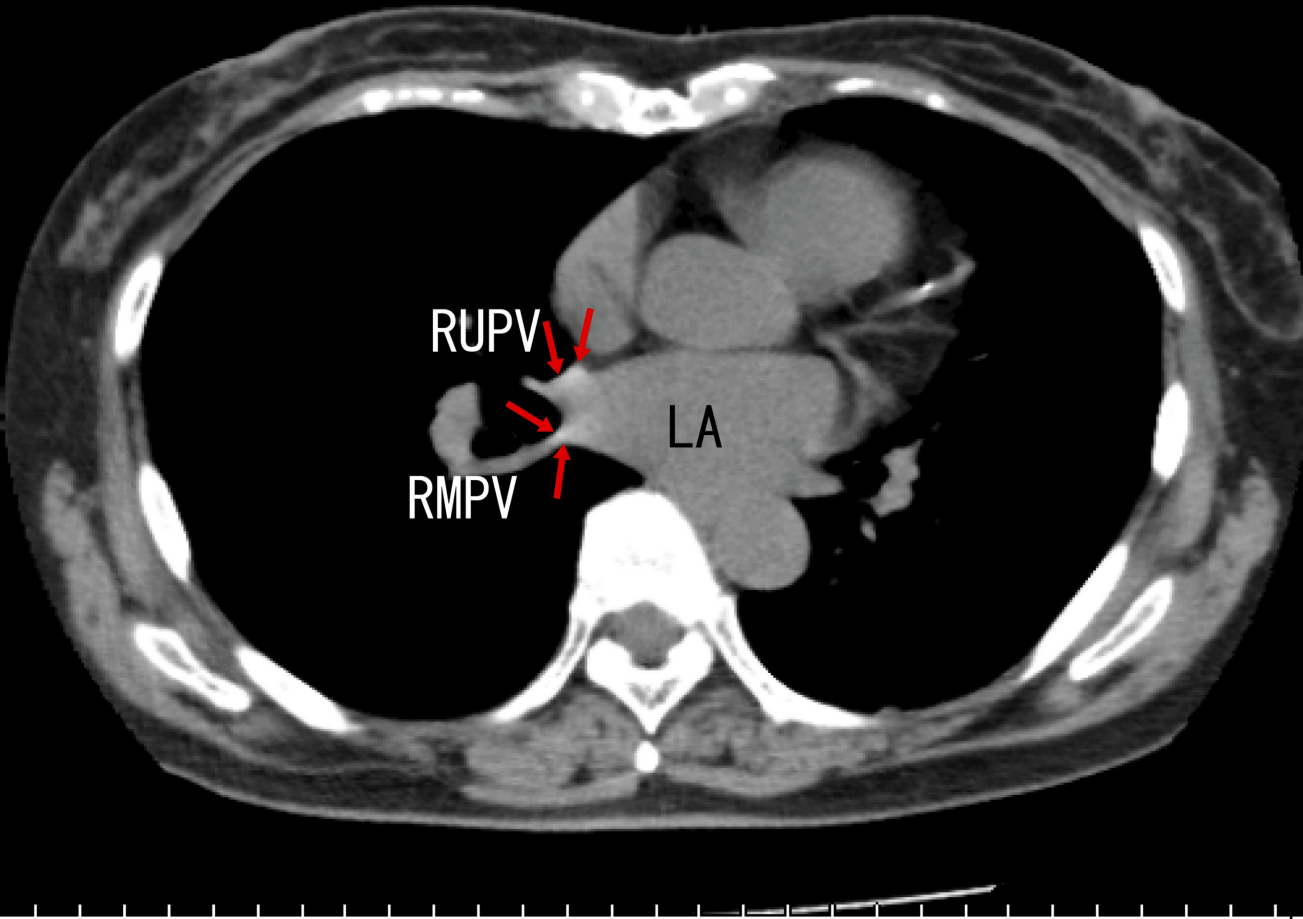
Axial plain computed tomography (CT) images Axial images depict calcification in the RUPV and right middle pulmopnary vein (RMPV) as white areas (arrows). LA: left atrium, RMPV: right middle pulmonary vein, RUPV: right upper pulmonary vein
